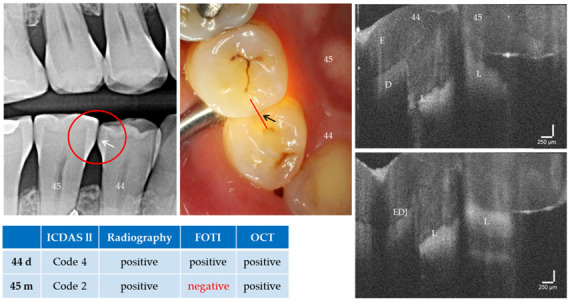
Teeth 44 (distal) and 45 (mesial). Detection of approximal caries lesions (L) with visual inspection, bitewing radiology, FOTI and OCT. The distal surface of tooth 44 was assessed as carious by all methods (arrows, L). In contrast, tooth 45 mesially was assessed as being sound with FOTI. In the OCT cross-sectional images the carious lesions are clearly visible on both teeth. Tooth 44 (ROI) shows no dentin involvement in the upper OCT image, whereas this can be suspected in the lower image. Both tooth surfaces showed no cavitation even with variable positioning of the OCT probe. *As a result of focusing on deeper structures, the tooth surface has flipped in the OCT images. Enamel (E), dentin (D), enamel-dentin junction (EDJ). The vertical scales are related to the refractive index n = 1.0 (see remark in Figure 4).*
